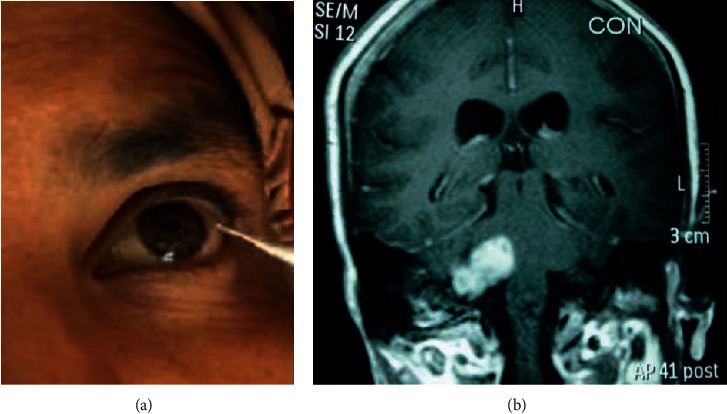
A 50-year-old female presenting with electric shock-like pain on the right side of the face for last 10 years. Patient had a history of multiple neurectomy of the infraorbital, mental, and inferior alveolar nerve with temporary relief followed by recurrence of symptoms. Corneal reflex was found absent. MRI revealed 2.2 × 2.2 × 2.7 extra-axial mass in the right cerebellopontine angle cistern suggestive of acoustic neuroma.
